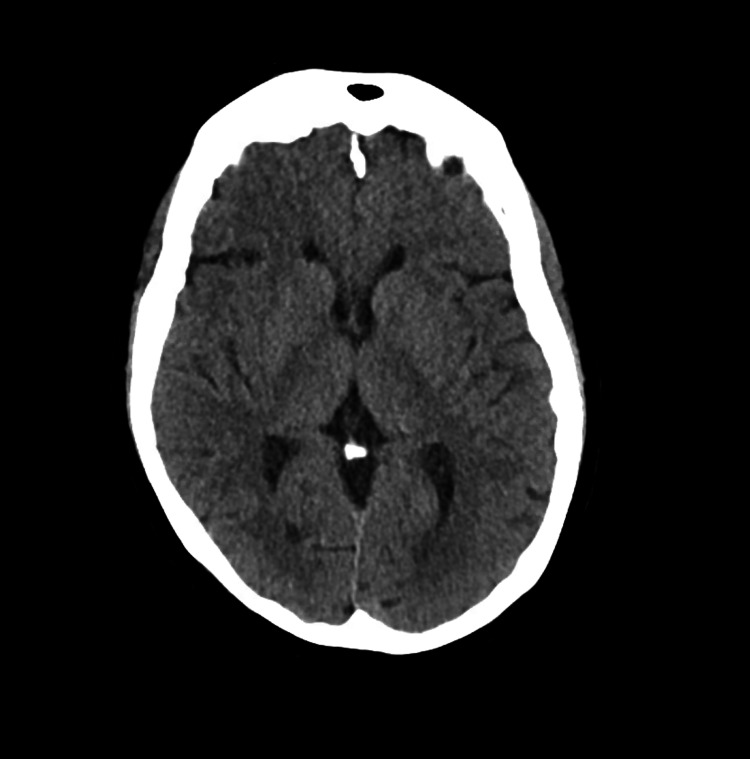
CT imaging of the head without intravenous contrast showing no evidence of any acute abnormalities.
